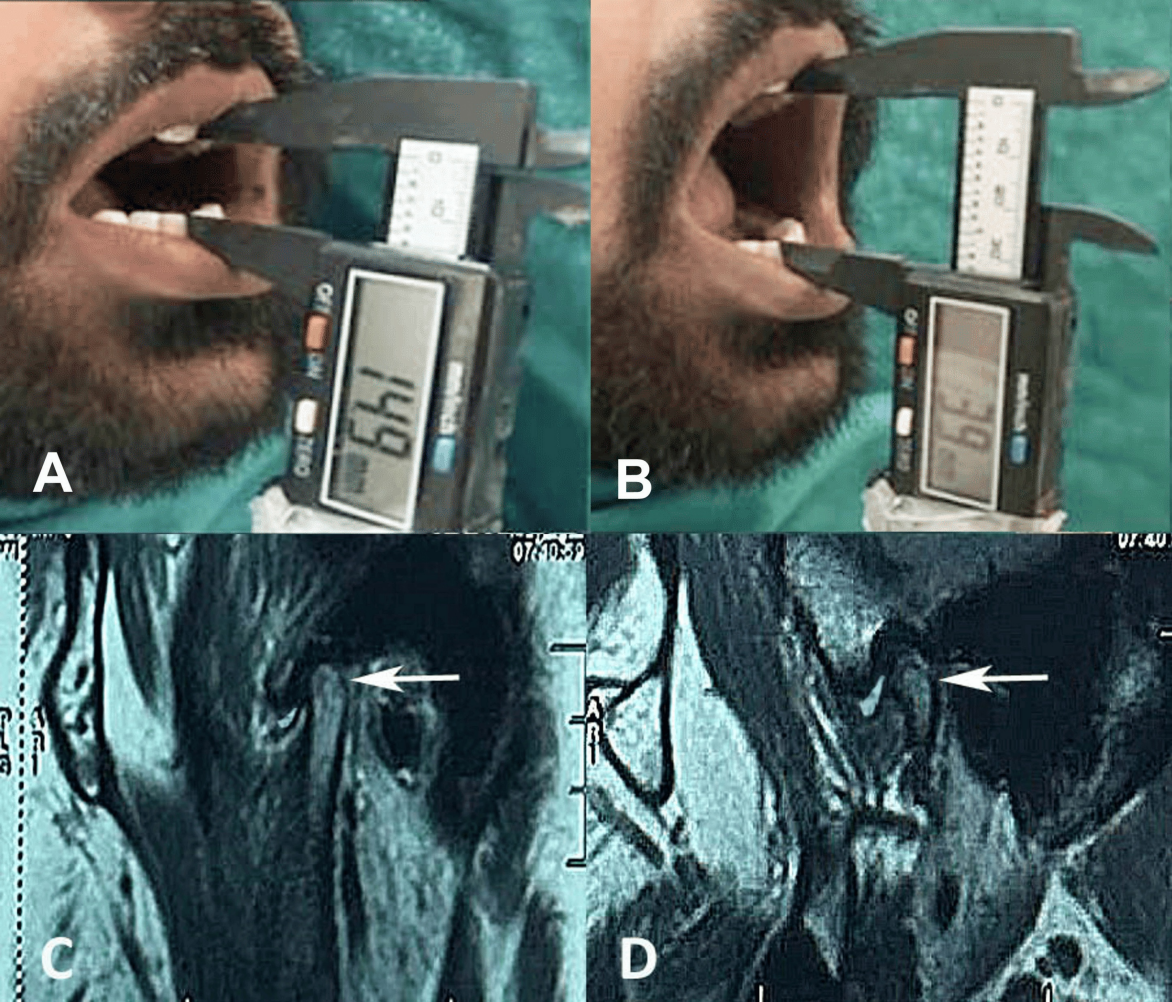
(A) Preoperative mouth opening (14.9 mm), (B) postoperative mouth opening (39 mm) at the three-month follow-up, (C) MRI scan of the closed mouth showing right temporomandibular joint effusion, and (D) MRI scan of the open mouth showing right temporomandibular joint effusion.
